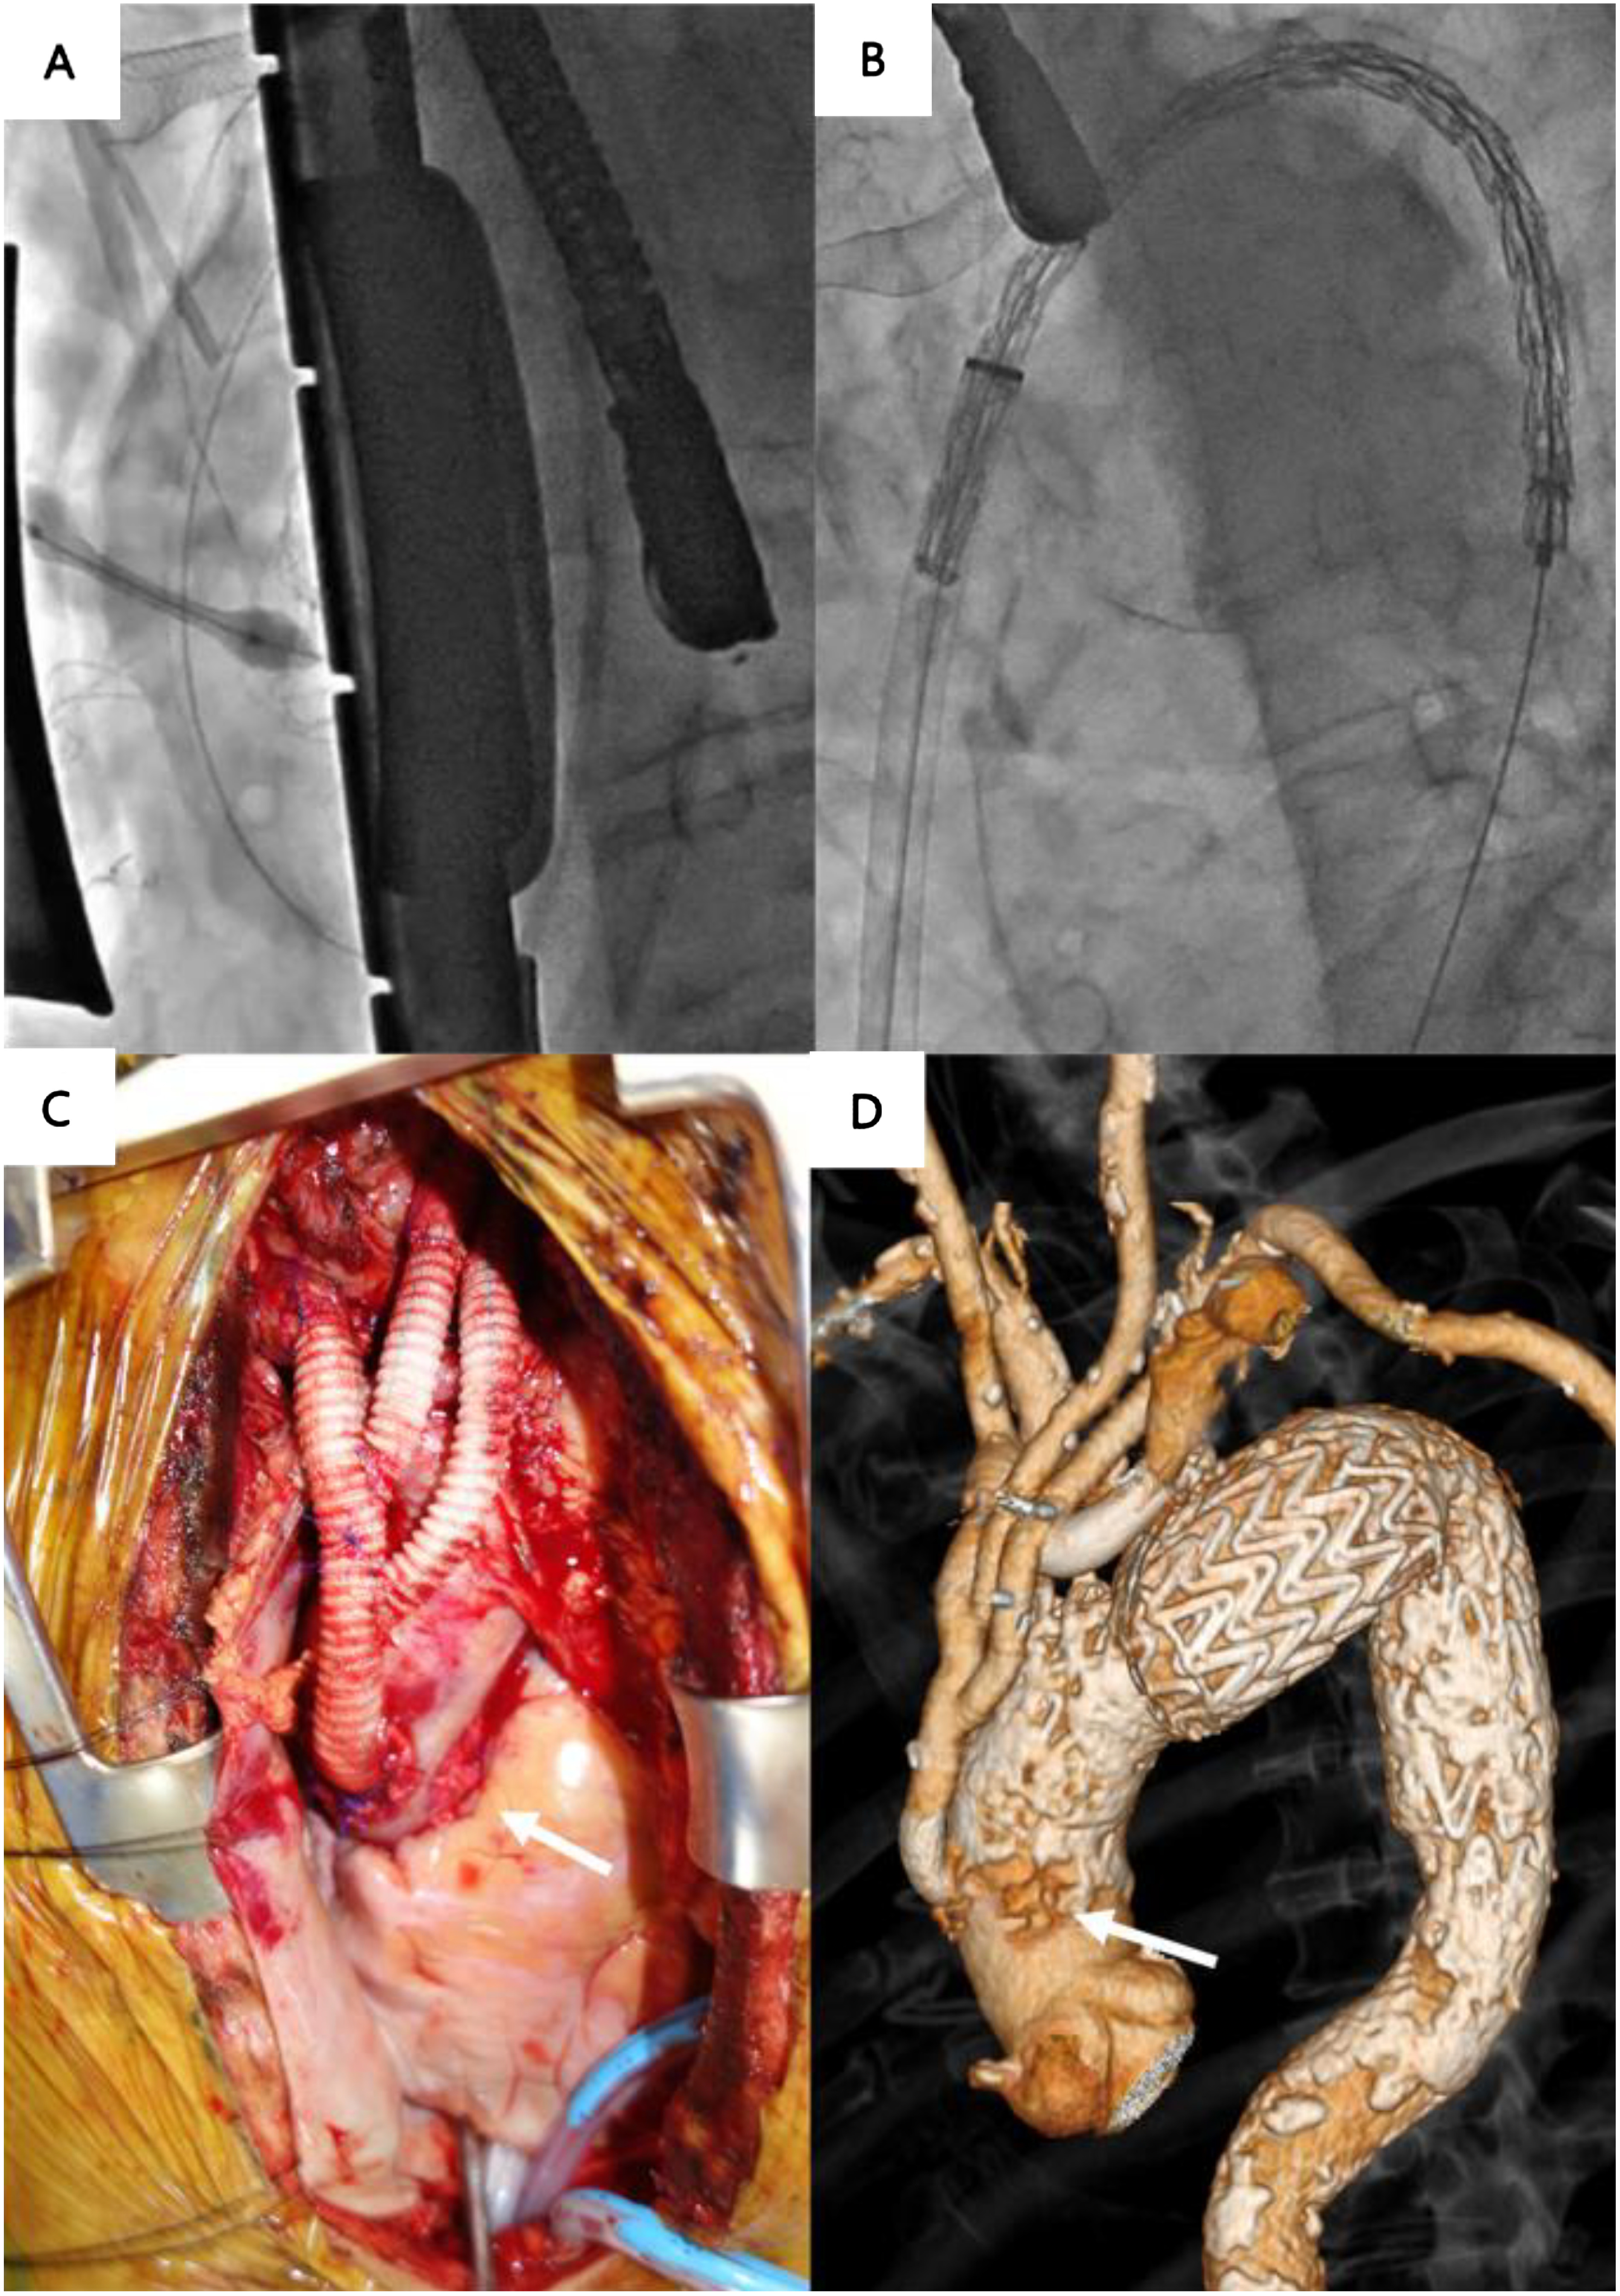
Fig. 3 (A) A VIABAHN VBX was placed into the proximal anastomosis. (B) A 24-Fr Dryseal sheath was placed, and conformable TAG was advanced in the ascending aorta. (C) Total debranching using the “real chimney technique.” The white arrow shows the device insertion point. (D) Postoperative computed tomography scans revealed successful exclusion of the aneurysm without an endoleak. The white arrow shows the device insertion point.
